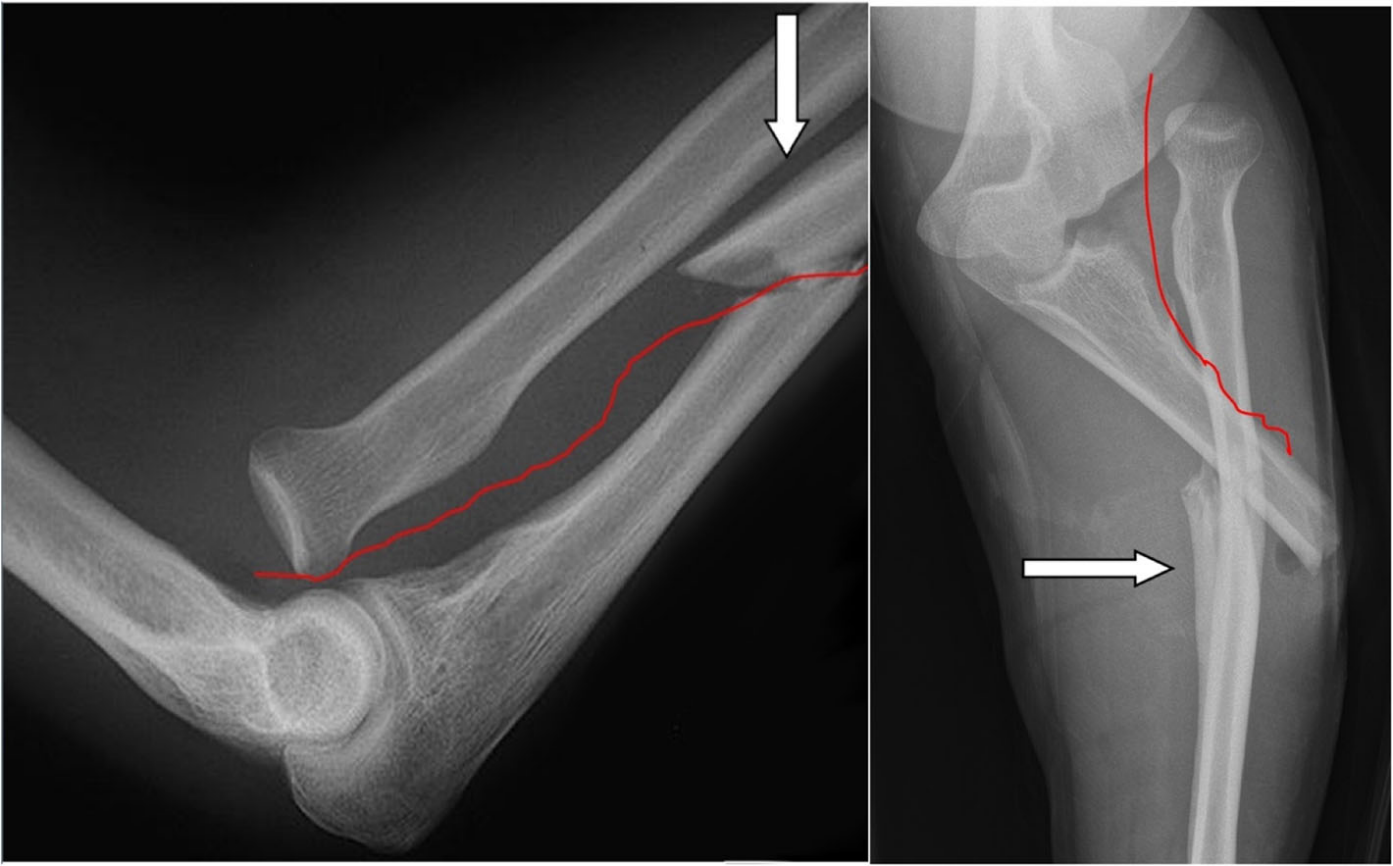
X-ray of a Monteggia fracture (Bado I) with potential injury of the proximal ligamentary structures between the ulna and the radius including the PRUJ until the end of the distal ulnar fracture (white arrow) in left: lateral and right: anteroposterior view
